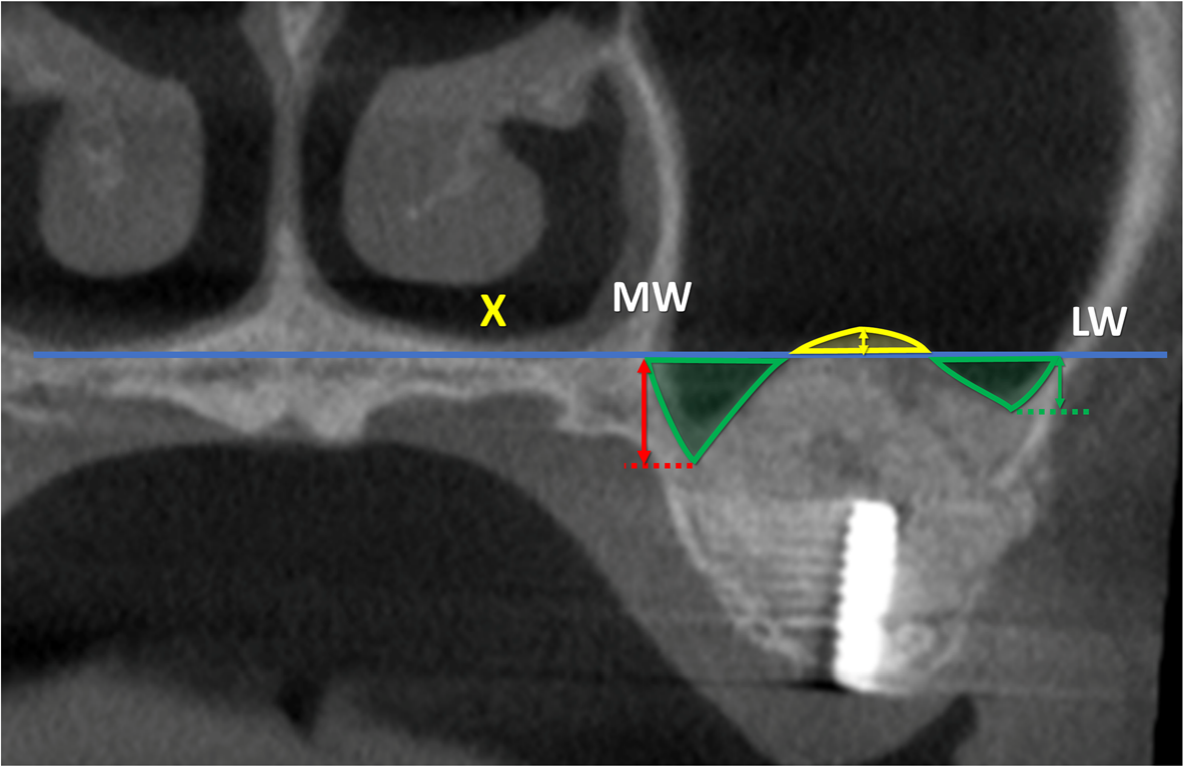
T2, coronal view of a no-membrane CBCT; X, line drawn following the floor of the nose; MW, medial wall of the sinus; LW, lateral wall of the sinus; Exceeding area (bordered in yellow), area above the line X filled with biomaterial/ bone tissue. Residual areas (bordered in green), the area below the line X not filled with biomaterial/ bone tissue. Floor augmentation heights at the lateral (green arrow), middle (yellow arrow), and medial (red arrow) aspects
